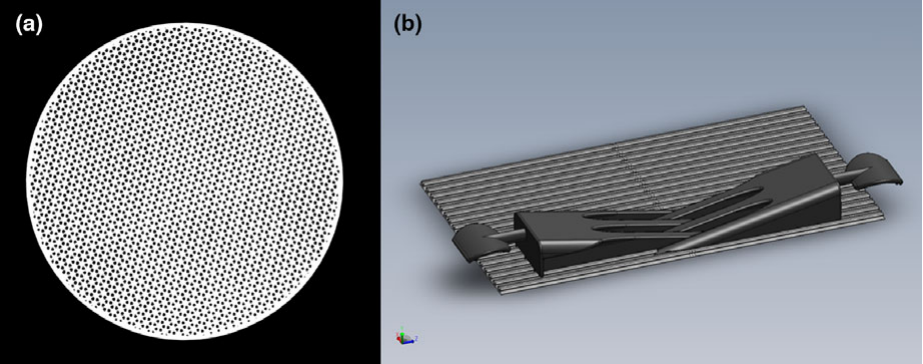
3D‐printed devices used for radiologic characterization. (a) A coronal slice of a CT image of the representative cylinder, and (b) 3D rendering of the CSI immobilization board to be used clinically.
